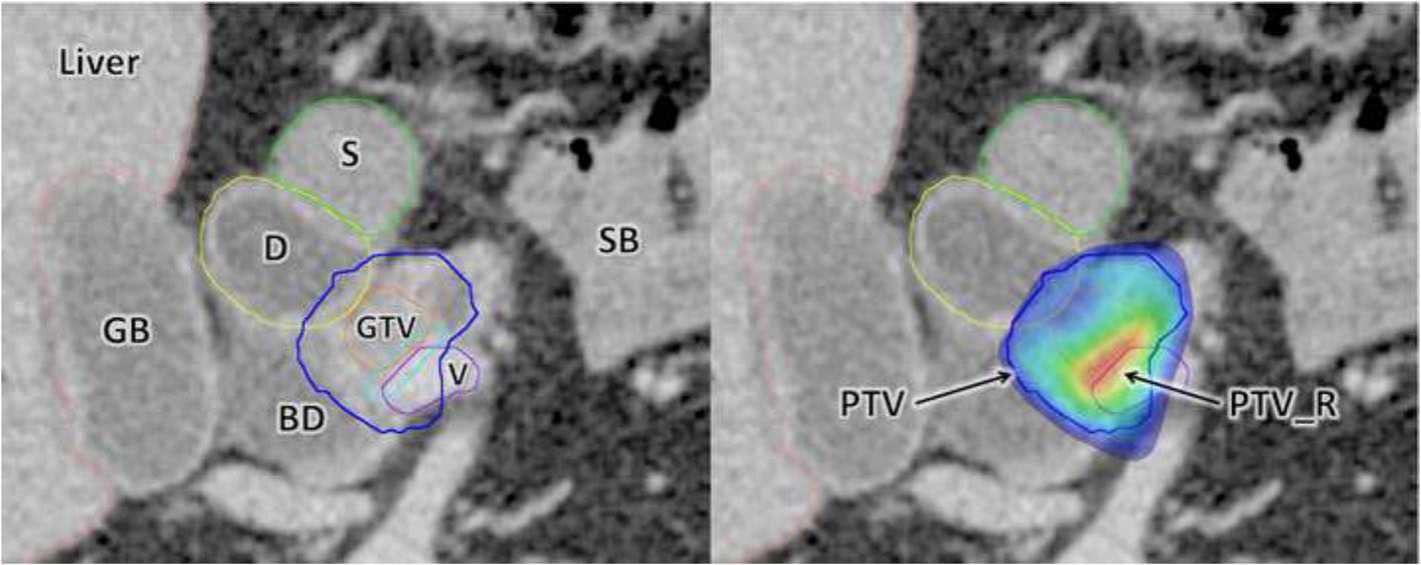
Axial contrast-enhanced CT of patient with borderline-resectable pancreatic cancer demonstrating SPARC radiotherapy planning. Left-hand image - delineated structures: (*clockwise from left*) GB = gall-bladder, D = duodenum, S = stomach, SB = small-bowel, V = vessel in contact with tumour, GTV = Gross Tumour Volume, BD = bile duct. Right-hand image - radiotherapy plan dose colourwash demonstrating dose levels delivered to PTV_R (*light blue contour*), PTV (*dark blue*) and PTV overlapping with duodenum
